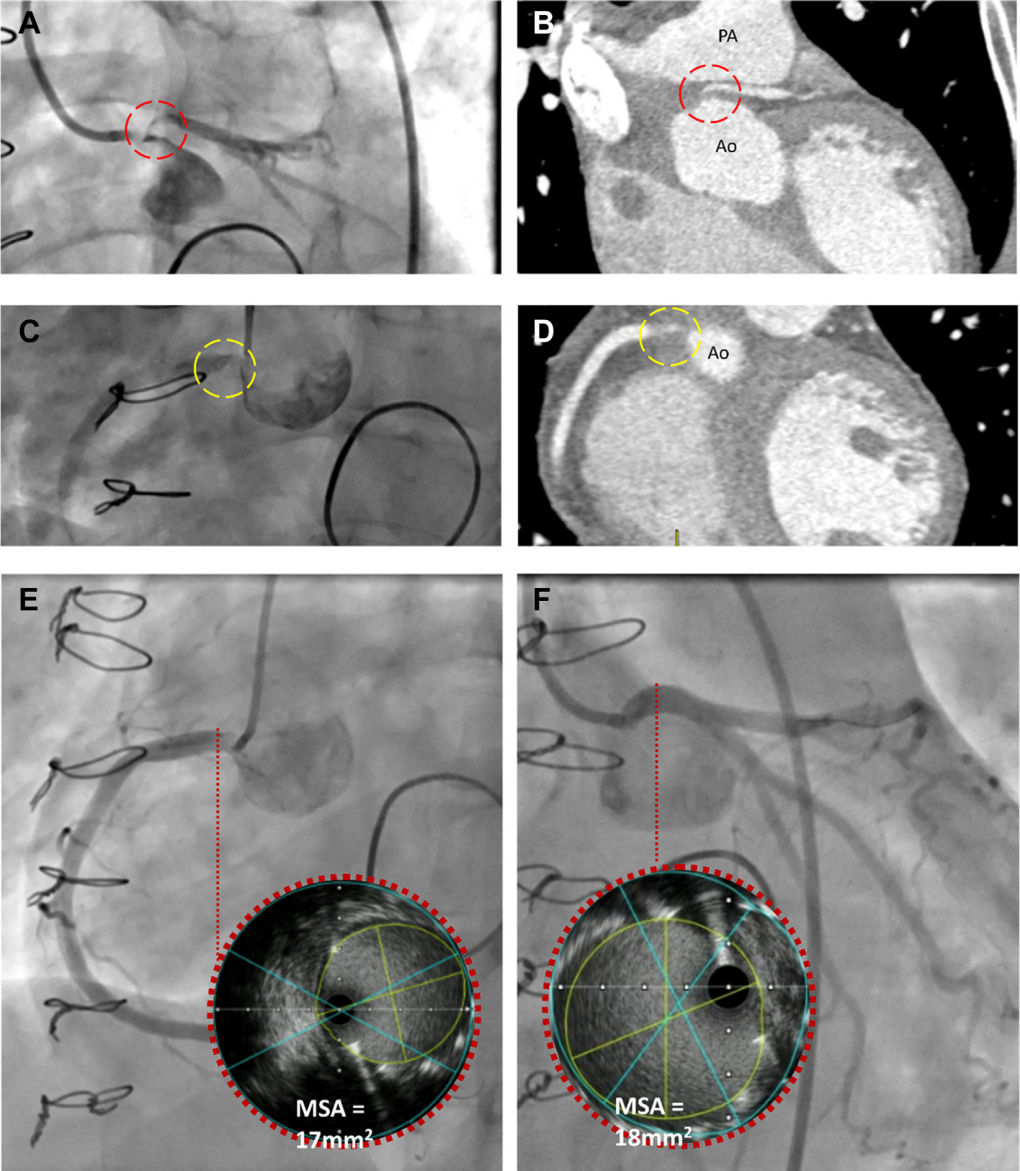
Severe Ostial Stenosis (A and C) Severe ostial stenosis of the left (red circle) and right coronary ostia (yellow circle) on invasive coronary angiography. (B and D) Respective findings on reconstructed computed tomographic coronary angiography. (E and F) Final angiographic result after successful deployment of drug eluting stents to the right (E) and left (F) coronary ostia with corresponding IVUS frames of MSA showing satisfactory deployment. Ao = aorta; IVUS = intravascular ultrasound; MSA = minimum stent area; PA = pulmonary artery.
